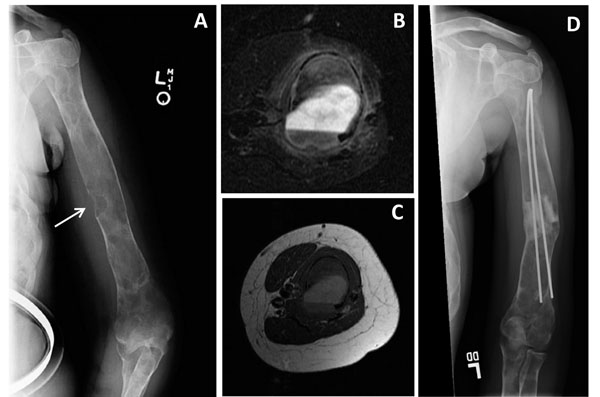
Aneurysmal bone cyst (ABC) of the upper extremity in fibrous dysplasia. This 16 year-old girl with McCune-Albright syndrome developed pain in the left humerus. A radiograph revealed a new lytic lesion that breeched the cortex (A, arrow). MRI revealed a fluid/fluid level within the lesion consistent with an ABC (B&C). The post-surgery radiograph shows a good result with the use of grafting material and flexible intramedullary rods (D).
